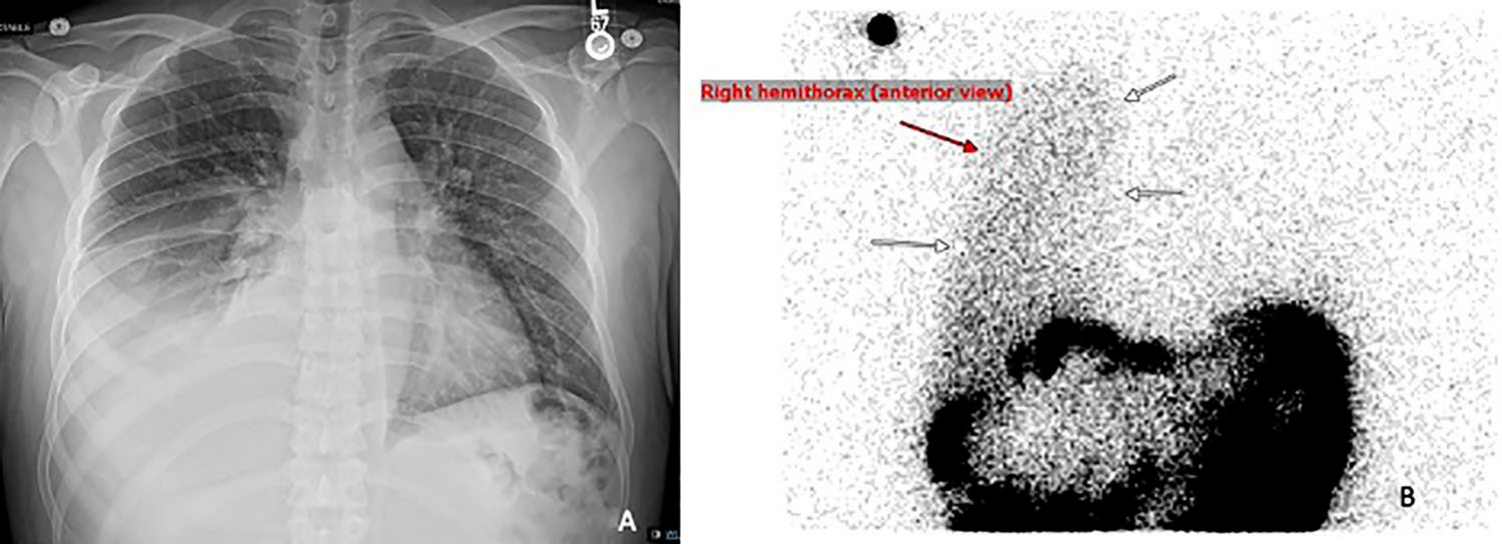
a chest X-ray. b Scintigraphy images indicating pleural-peritoneal fistula
